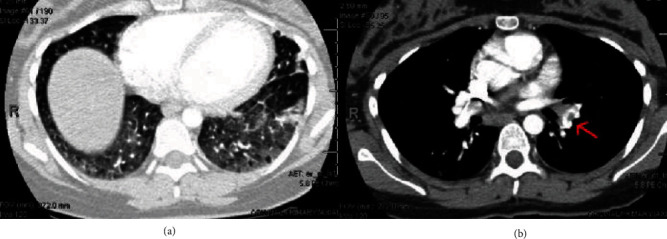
Computed tomography of the chest demonstrating diffuse ground-glass opacities (a) throughout both lungs, which can be seen with COVID-19 pneumonia. Acute pulmonary emboli (b) identified in segmental branches of lingula and left inferior lobar pulmonary artery extending into segmental branches of left lower lobe, with filling defect noted by red arrow.
